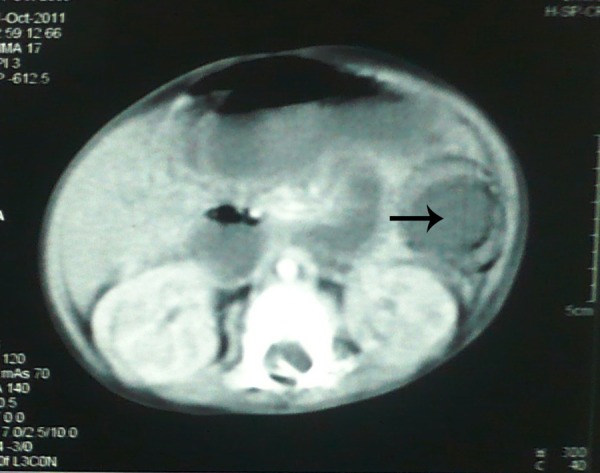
Figure 2: CT scan showing a hypo-dense intraluminal bowel mass.
